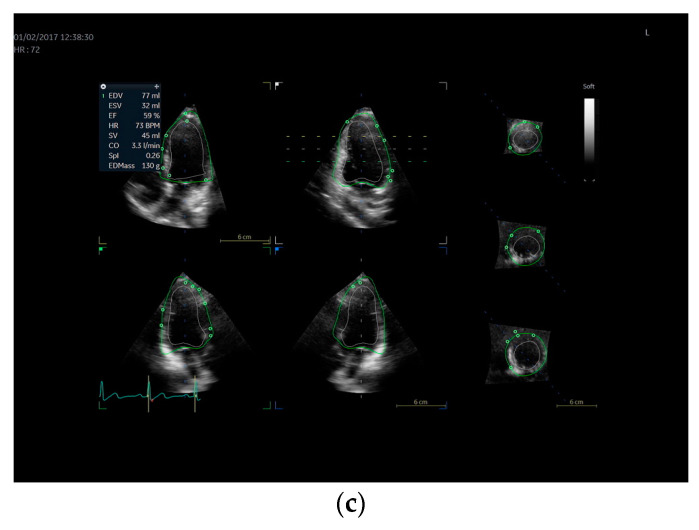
Exemplary 3D echocardiography images of a single participant with measurements made at (a) follicular, (b) ovulation, and (c) luteal phases of the menstrual cycle.
